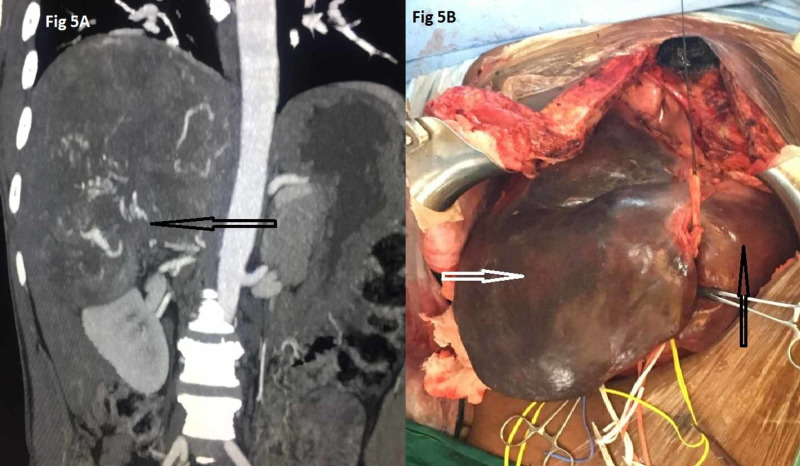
A: CT image showing right adrenal mass with infiltration of right kidney and indistinct planes with liver and extensive tumor vascularity. B: Intraoperative picture of the same case where median sternotomy was done for supra diaphragmatic IVC control and veno-venous bypass. Black arrow indicates left lobe of liver and white arrow indicates adrenal mass with extensive infiltration of right lobe of liver. IVC, inferior vena caval
